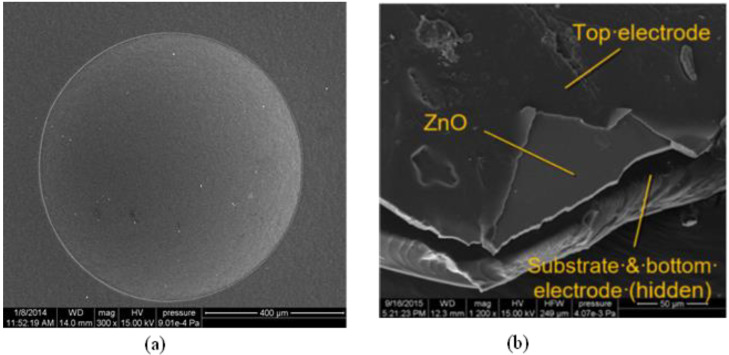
SEM images of the ZnO piezoelectric film self-focusing ultrasonic transducer (a) spherical cap and (b) ZnO film and electrode.
